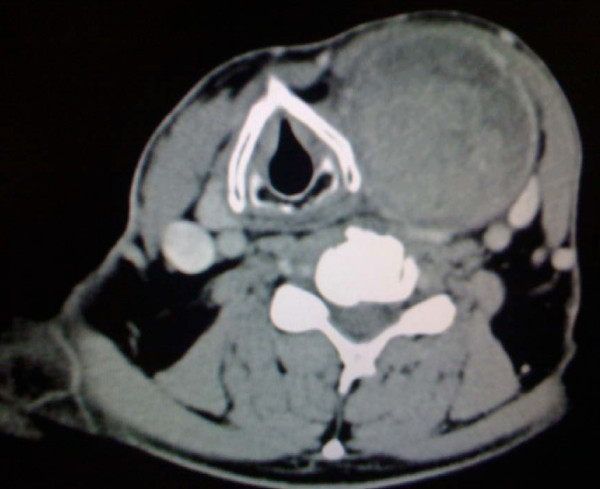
Axial computed tomography (CT) image showing the displacement of the larynx to the right and the inhomogeneous mass on the left.
